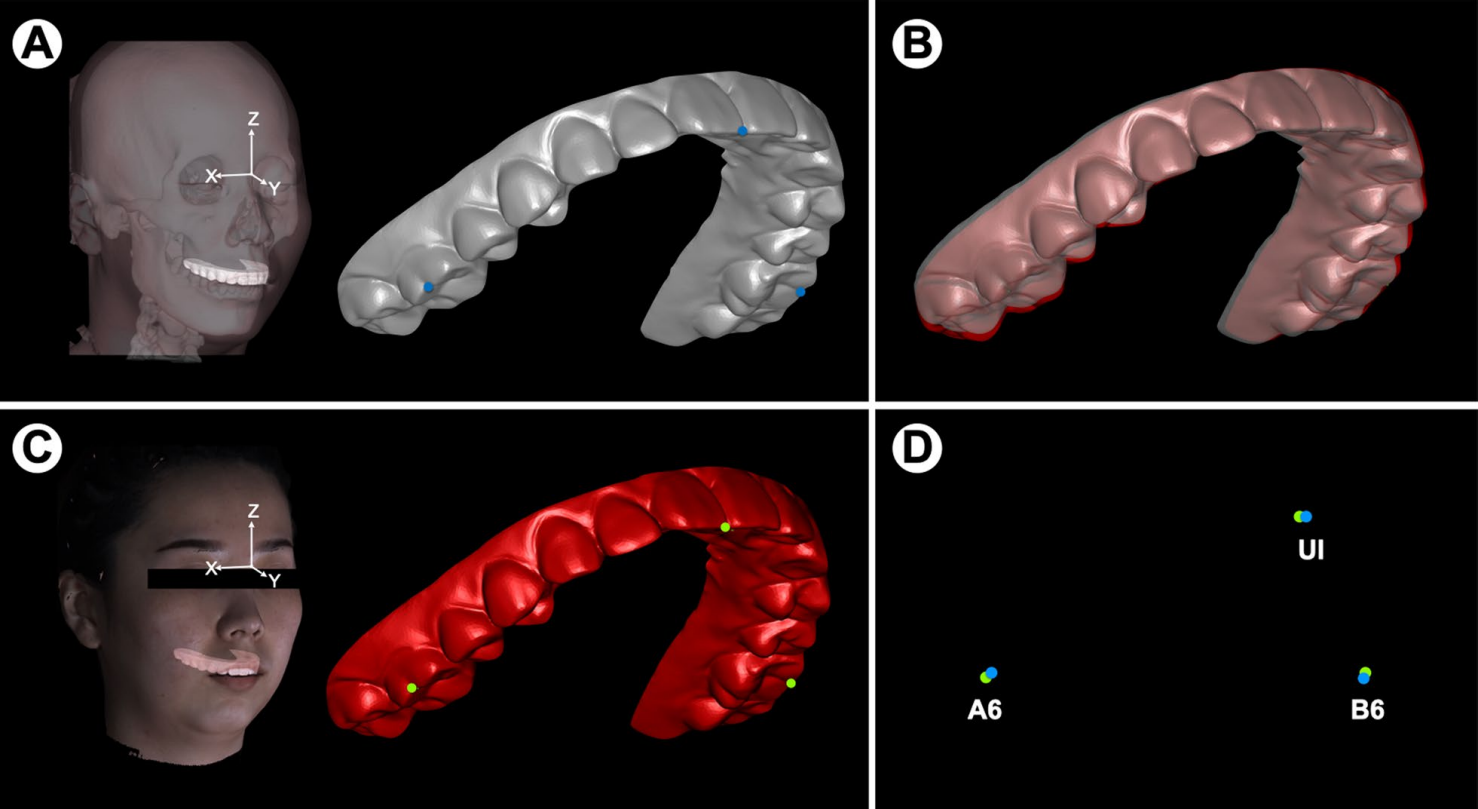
Assessment of accuracy. A 3D skull reconstructed from CT data was registered to PHOTO1 with the facial global reference system. The coordinate values of the pre-selected landmarks (blue) on the maxillary dentition were recorded in the CT-integrated position (grey), and the orientations (pitch, roll, yaw) of the maxillary dentition were all set as 0°. B By selecting the same region on the dentition, the CT-integrated dentition (grey) with the landmarks “glued” on it was registered to the CAD/CAM facebow-integrated dentition (red). C The coordinate values of the landmarks in the position of the CAD/CAM facebow-integrated dentition (green) were recorded. D The rotational deviations between the CT-integrated and facebow-integrated dentitions and the translational deviations as represented by differences between the coordinates of the landmarks in the CT-integrated dentition (blue) and the facebow-integrated dentition (green) were automatically recorded
